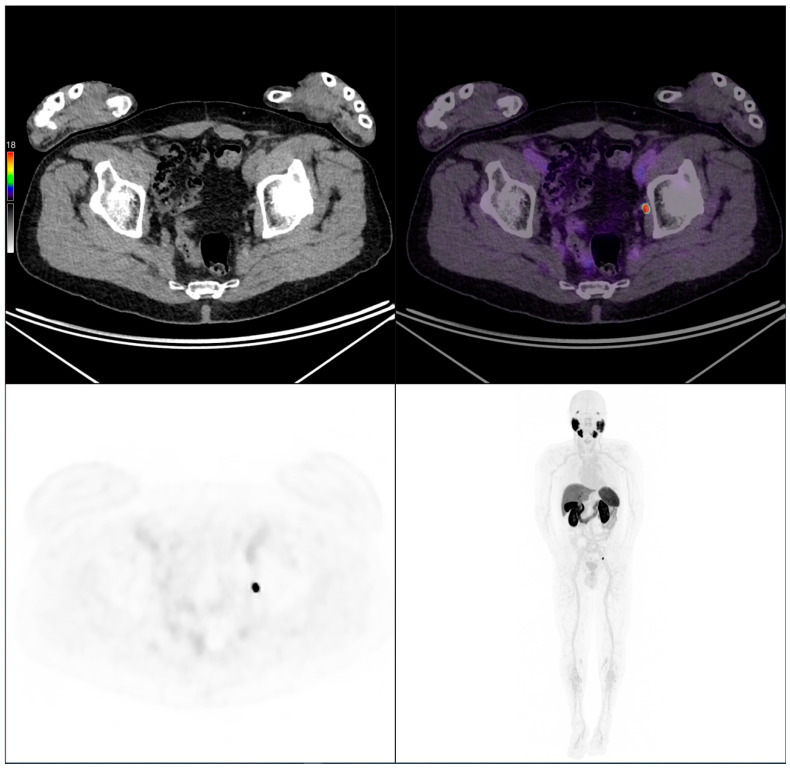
PSMA PET/CT scan of an 83-year-old patient with biochemical recurrence (PSA: 0.4 ng/mL). Initially, the Gleason score was 4 + 3 = 7, pT3 pN0 M0, and treated with radical prostatectomy. Imaging performed following intravenous administration of 208 MBq of [^18^F]PSMA-1007 revealed a left obturator lymph node metastasis consistent with a local recurrence.
